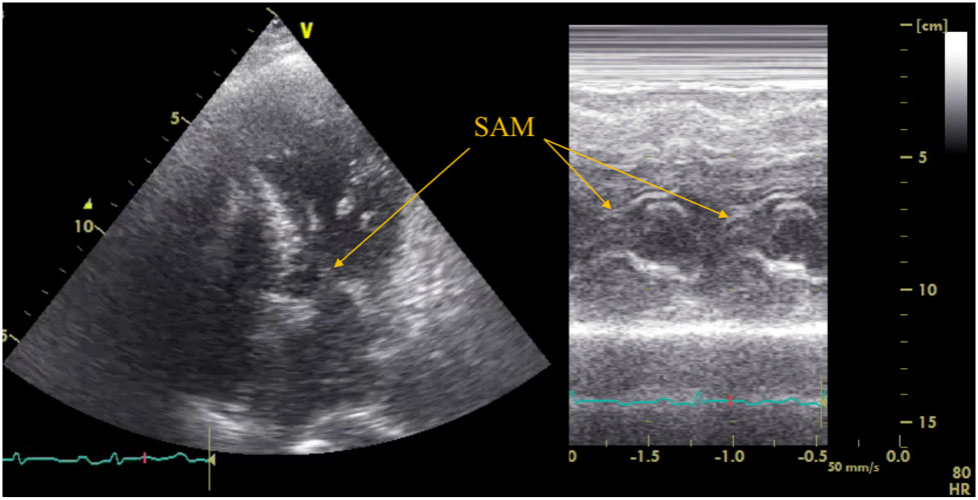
Echocardiography of left ventricular outflow tract obstruction with systolic anterior motion and basal hyperkinesia. LVOT, left ventricular outflow tract; SAM, systolic anterior motion. *Note*: Apical five chamber view (left) and M-mode of SAM in left parasternal long axis view (right) of echocardiography.
